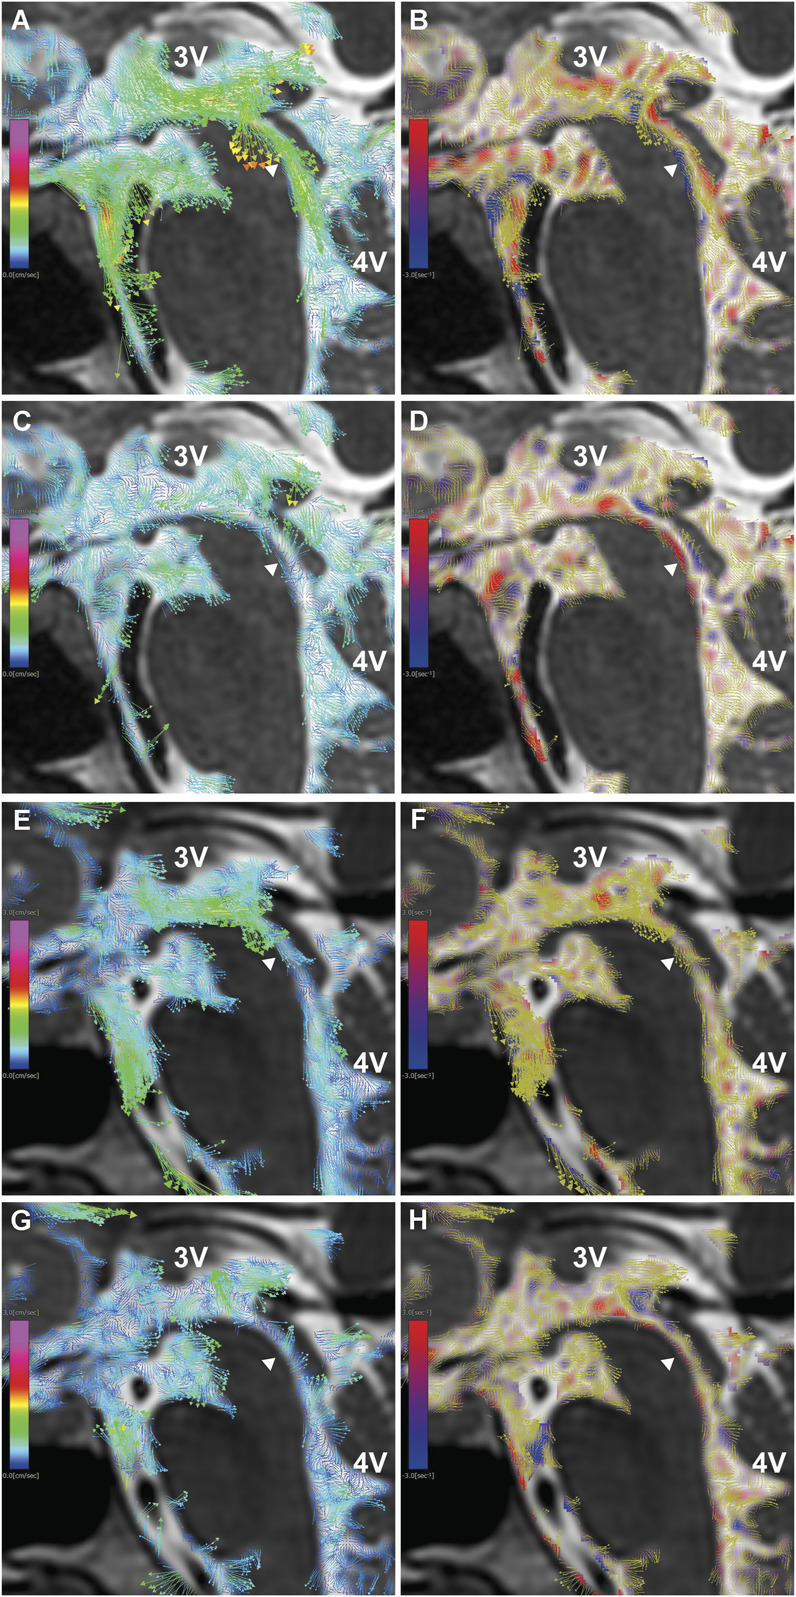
Normal CSF dynamics on 4D flow MRI. 4D flow MRI findings in a healthy 25-year-old man A-D, and a healthy 48-year-old man E-H. A, C, E, and G, Velocity maps from 2D sagittal views, with colored arrows representing CSF velocity vectors. B, D, F, and H, Vorticity maps from 2D sagittal views, showing clockwise vortices in blue, counterclockwise vortices in red, and overlaid velocity vectors in yellow. CSF flow from the 3V into the 4V through the cerebral aqueduct (white arrowhead) was observed. In addition, a short episode of reversed, high-velocity flow from the aqueduct back into the 3V occurred during the same cardiac cycle. During this reversed flow, prominent clockwise (blue) and counterclockwise (red) vortices were observed in the posterior 3V, located behind the interthalamic adhesion (Massa intermedia). 2D, 2-dimensional; 3V, third ventricle; 4D, 4-dimensional; 4V, fourth ventricle; CSF, cerebrospinal fluid.
